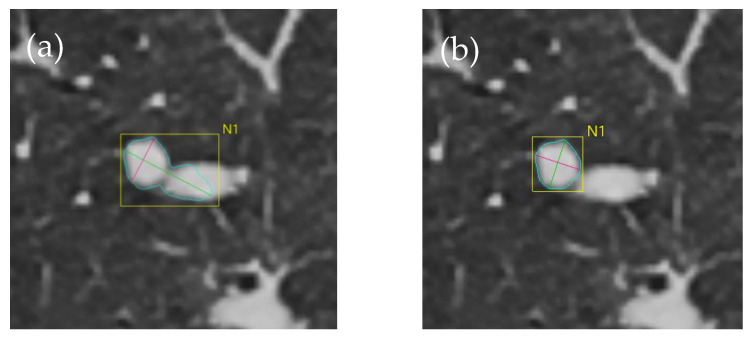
A complicated situation where the nodule partially blended together with blood vessels in certain CT slices. This caused the delineation to be elongated and partially incorrect, where both the nodule and parts of the blood vessel (on the right) were included in the initial estimate made by the semi-automatic algorithm ((a); volume: 431 mm^3^, dimensions: 11.2 × 7.5 mm; blue-colored lines represent the longest diameter). The reviewers adjusted the delineation to accurately depict the size of the nodule ((b); reviewer 3 after adjustments; volume: 142.8 mm^3^, diameter: 7.6 × 7.3 mm).
